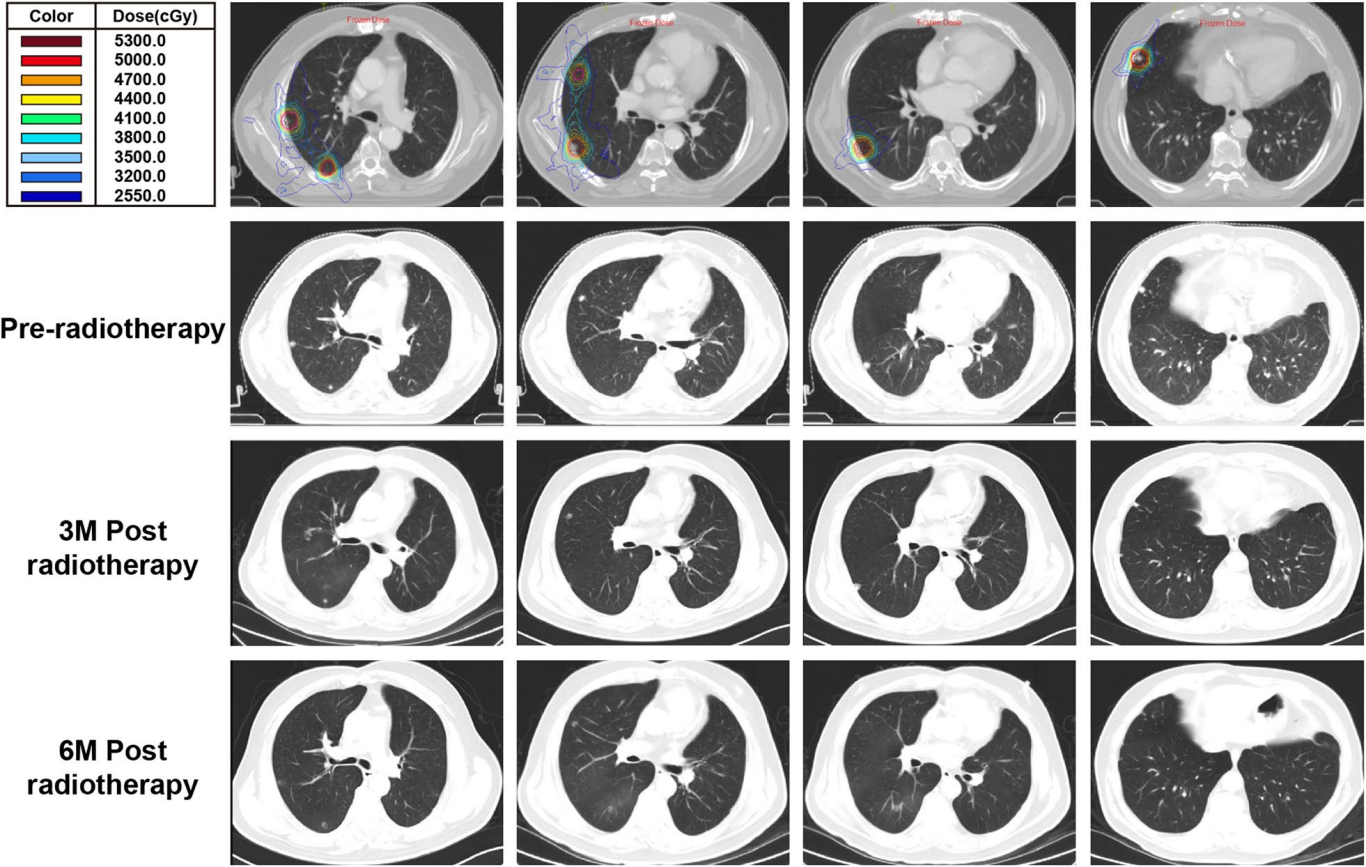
A typical patient for combination PD-1 inhibitor and chemoradiotherapy.The 4 metastatic lesions in right lung received radiation with median dose of 60 Gy (range 50–70 Gy).CT images were showed at pre-radiation, 3 months after the completion of radiation and 6 months after the completion of radiation
